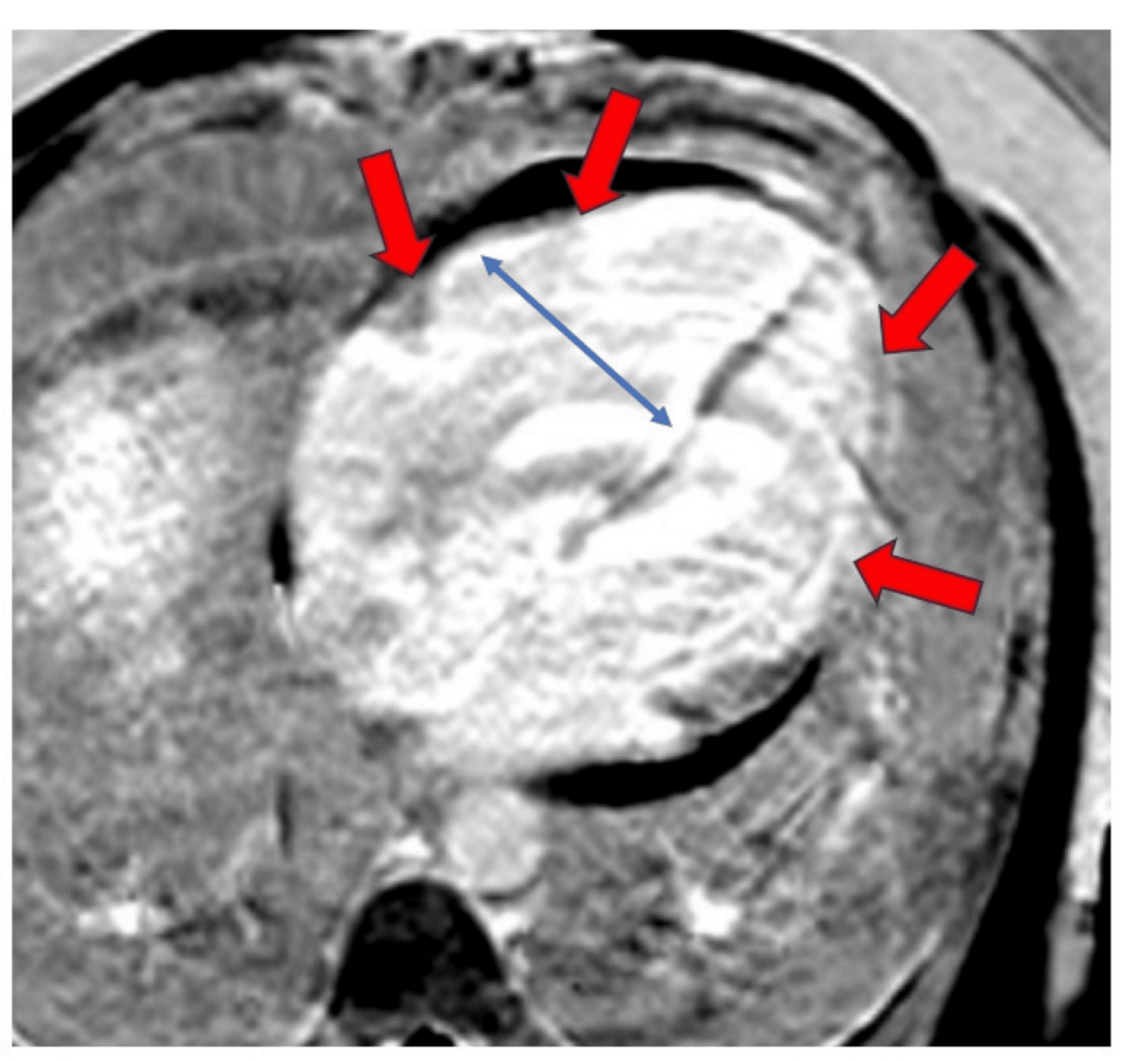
Cardiac MRI reveals late gadolinium enhancement in both the right and left ventricles (red arrow), along with dilation of the right ventricle (blue double-sided arrow) and “D-septum,” concerning for septal flattening in diastole, suggestive of right ventricular pressure overload.
